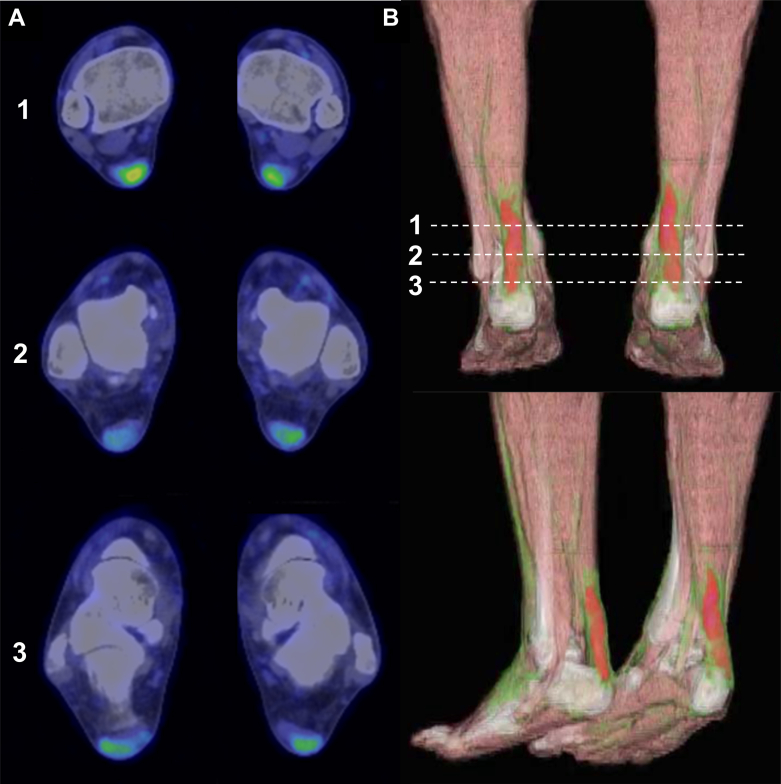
Metabolic Activity of Achilles Xanthomas on FDG-PET/CT in the Son FDG-PET/CT showing intense bilateral tracer uptake within the Achilles tendons, consistent with active inflammatory xanthomas. FDG-PET/CT = ^18^F-fluorodeoxyglucose positron emission tomography/computed tomography.
